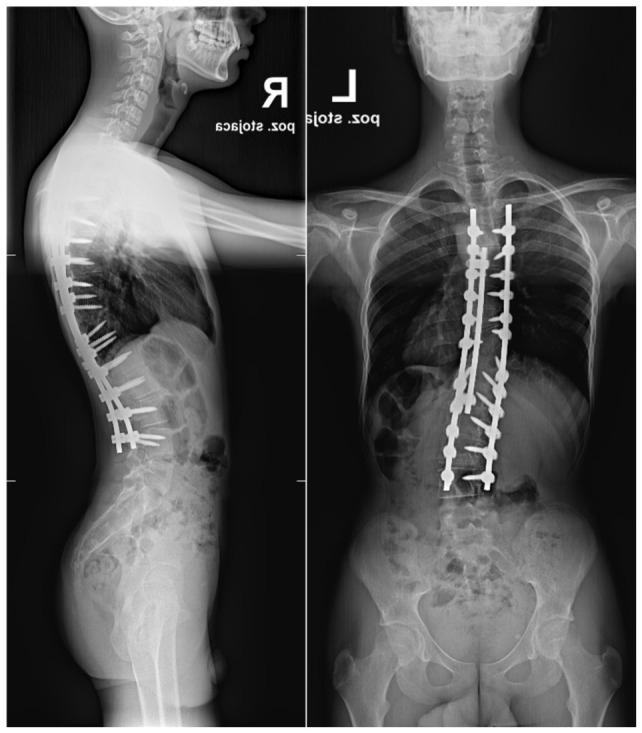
Standing posteroanterior and lateral full-spine radiographs obtained at a 2-year of follow-up.
